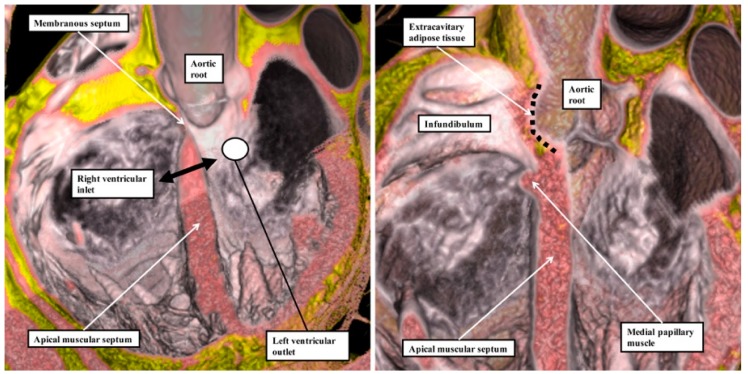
These images were prepared by virtual dissection of a cardiac computed tomographic dataset. The left-hand panel shows that the basal and inferior part of the ventricular septum interposes between the inlet of the right ventricle and the subaortic outlet of the left ventricle. The right-hand panel shows that the infundibulum of the right ventricle is a free-standing myocardial sleeve. By virtue of the presence of this free-standing sleeve, there is no “outlet septum” in the normal heart.
